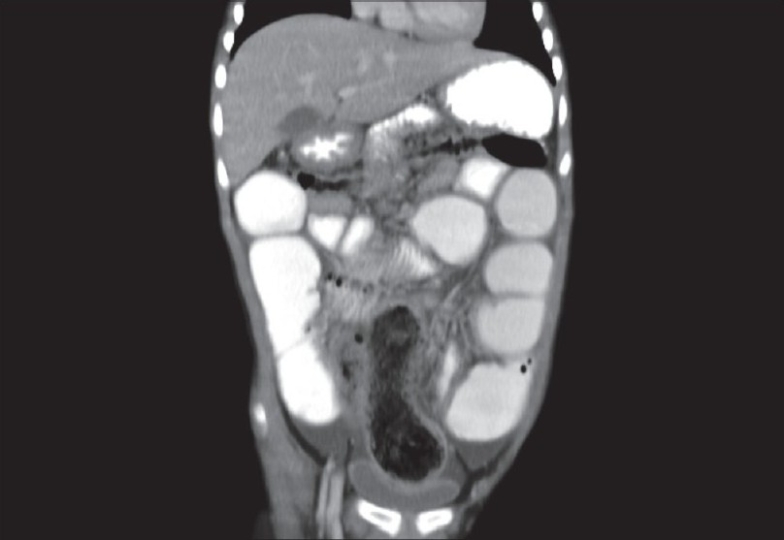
Coronal view on CT with bezoar
